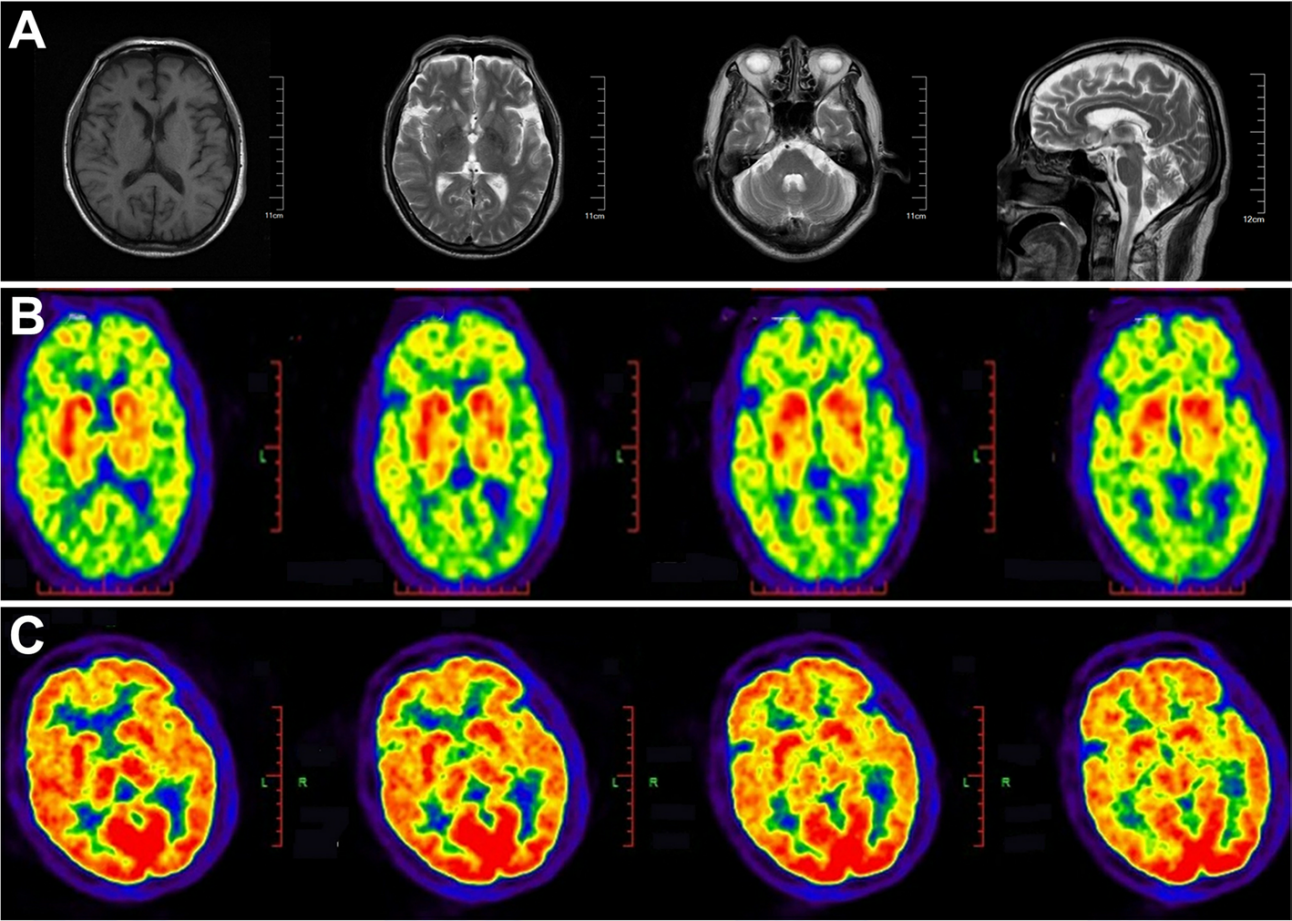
A Images of the patient’s MRI. Brain MRI showed multiple ischemic foci without brain stem or cerebellar atrophy. B Images of the patient’s ^11^C-CFTPET/CT. ^11^C-CFTPET/CT scan showed low metabolism of the left caudate nucleus and bilateral putamen. C Images of the patient’s ^18^F-FDGPET/CT. ^18^F-FDGPET/CT scan showed symmetrical low metabolism of bilateral frontal-parietal lobes
